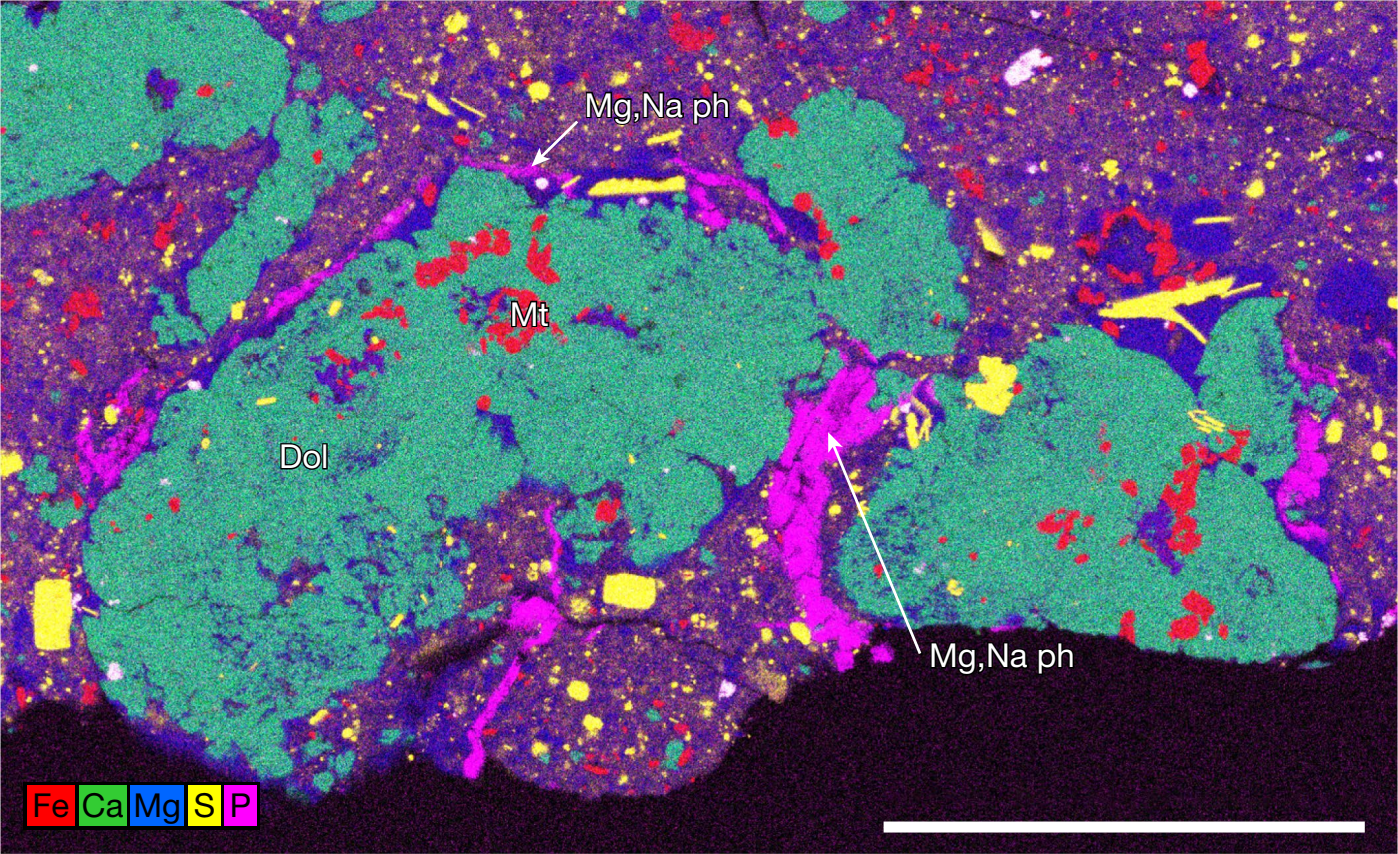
Mg,Na phosphate occurs as sheets and veins. Mg,Na phosphate occurs with Mg phyllosilicates largely free of micron-sized sulfide inclusions. The Mg,Na phosphate–Mg phyllosilicate veins rim large dolomite (Dol) grains that include magnetite (multielement Kα X-ray map; sample no. OREX-803085-100). Scale bar, 200 μm.
